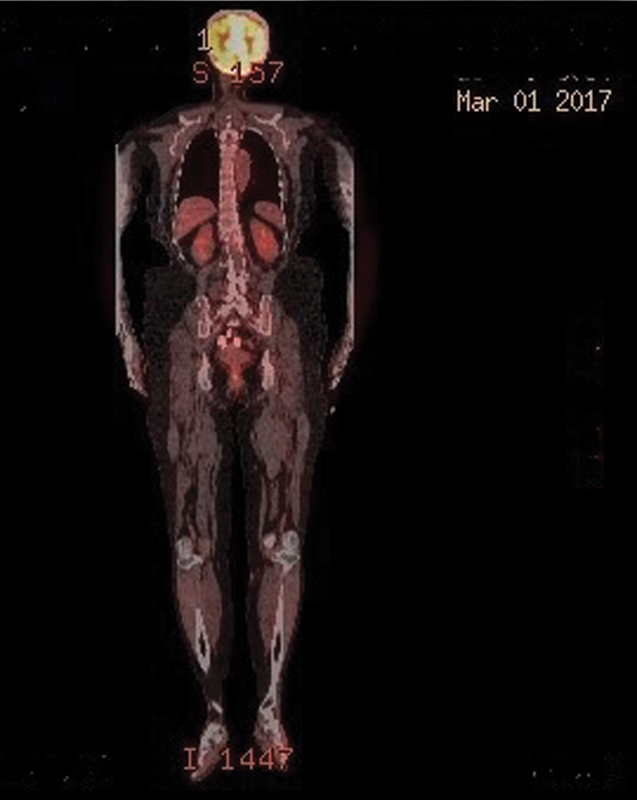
Complete remission after immunochemotherapy was showed on uterine and crural region on FDG-PET/CT (SUV: 2). CT, computed tomography; FDG, fluorodeoxyglucose; PET, positron emission tomography.
